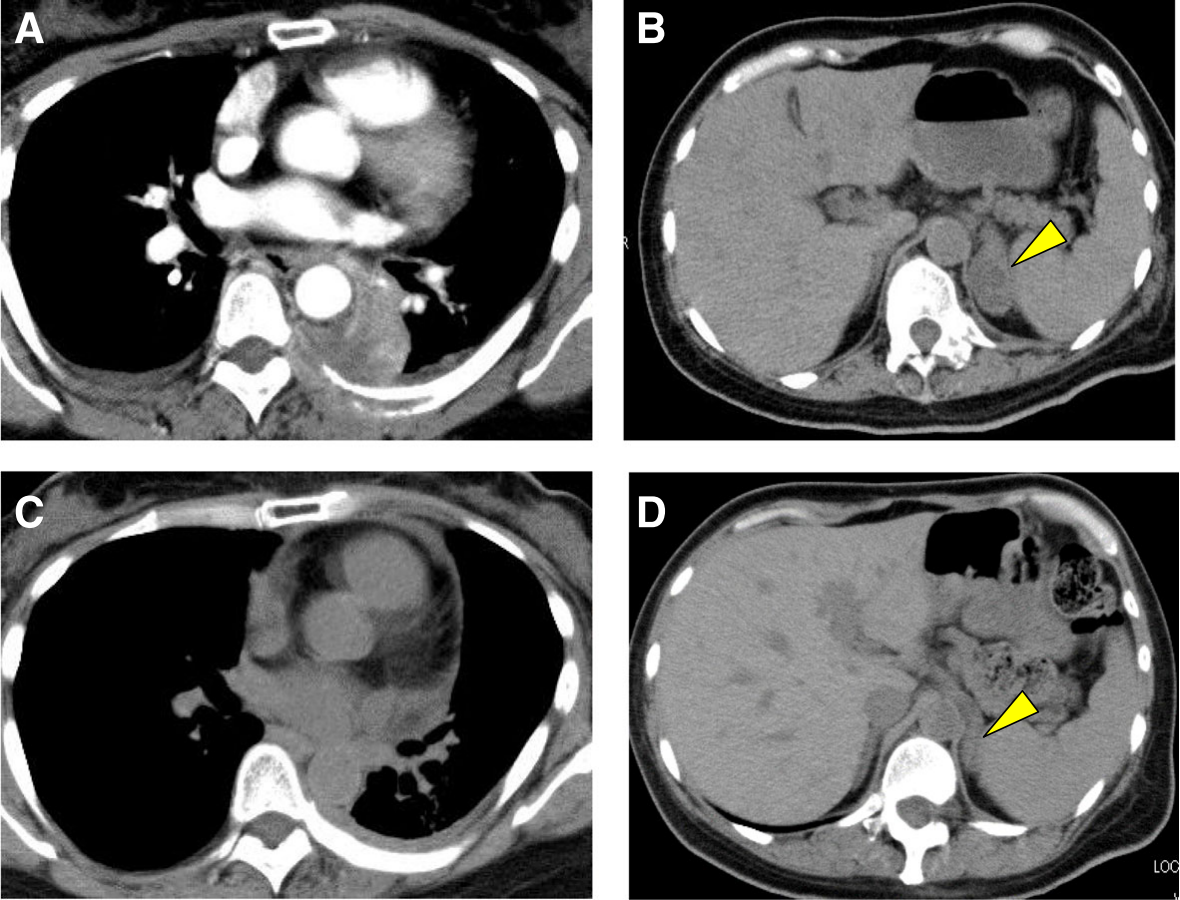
Computed tomography findings before and after treatment with alectinib. A computed tomography scan before treatment revealed (a) a solitary tumor in the lower lobe of the left lung and (b) a left adrenal metastasis (*arrow*). A computed tomography scan 3 months after commencing treatment revealed (c) a dramatic reduction in tumor size and (d) almost no presence of metastases in the left adrenal gland (*arrow*)
